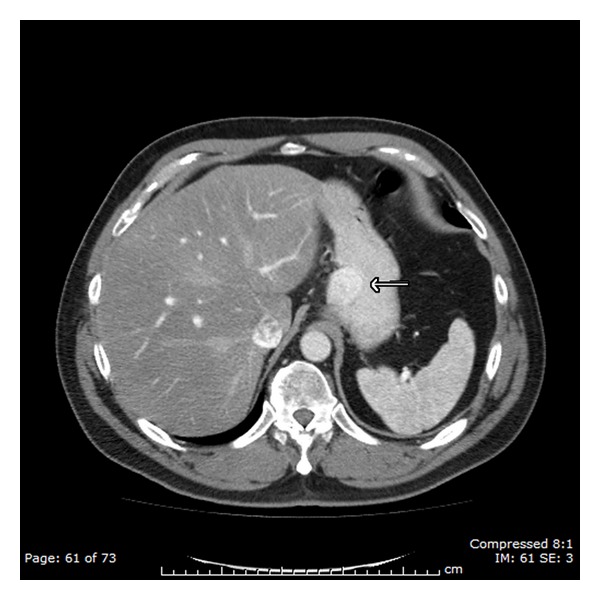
Axial CT scan of the abdomen, demonstrating a 36 × 34 mm submucosal circumscribed lesion on the lesser curvature of the stomach (arrow).
